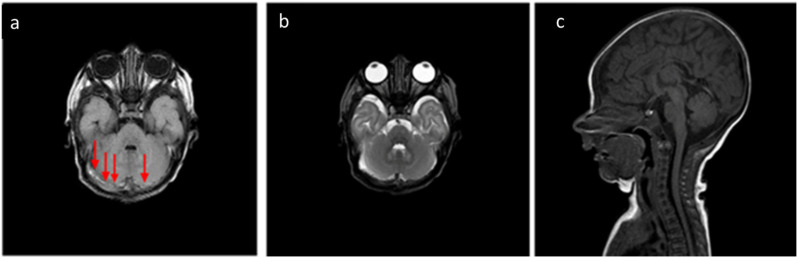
In the same patient in Figure 2, (a) FLAIR T2-weighted sequence in the axial plane at the level of the posterior cranial fossa—the subdural hematomas described in the bilateral retrocerebellar region (solid arrow) also appear hyperintense in this sequence, as in the late subacute phase (extracellular methemoglobin). (b) FSE T2-weighted sequence on the axial plane at the same 3a level—the known hematomas are difficult to appreciate in relation to the spontaneous hyperintensity. (c) SE T1-weighted in the midsagittal plane—the resolution of the hematomas described in Figure 2 and Figure 3 is observed in the follow-up examination.
